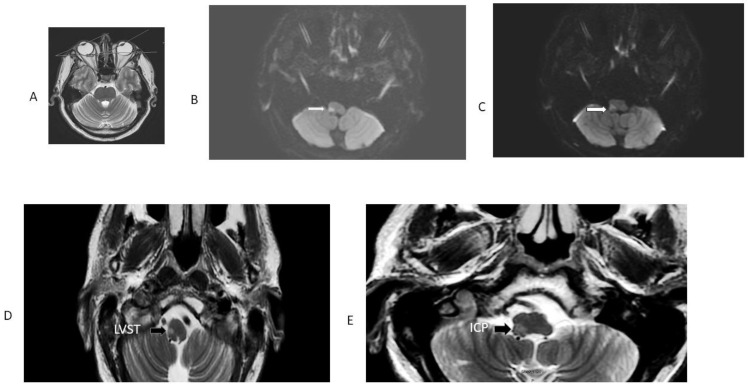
MRI patient 9, Table 1. This patient had an initially false-negative MRI, the Figure shows the repeat MRI 48 h after initiation of symptoms. Note radiographic conjugate horizontal deviation of the eyes toward the right, in the same direction of OLD in a T2 axial MRI (panel (A)). Panel (B) is an axial DWI; it shows restricted diffusion involving the right LVST and the CSCT, responsible for severe right-side truncal and limb ataxia. The adjacent panel (C) is a 3 mm rostral axial DWI section at the level of the right inferior cerebellar peduncle, which explains OLD. The ADC map confirmed diffusion restriction. Panels (D) and (E) are T2 FLAIR images showing the same structures with greater anatomic resolution.
